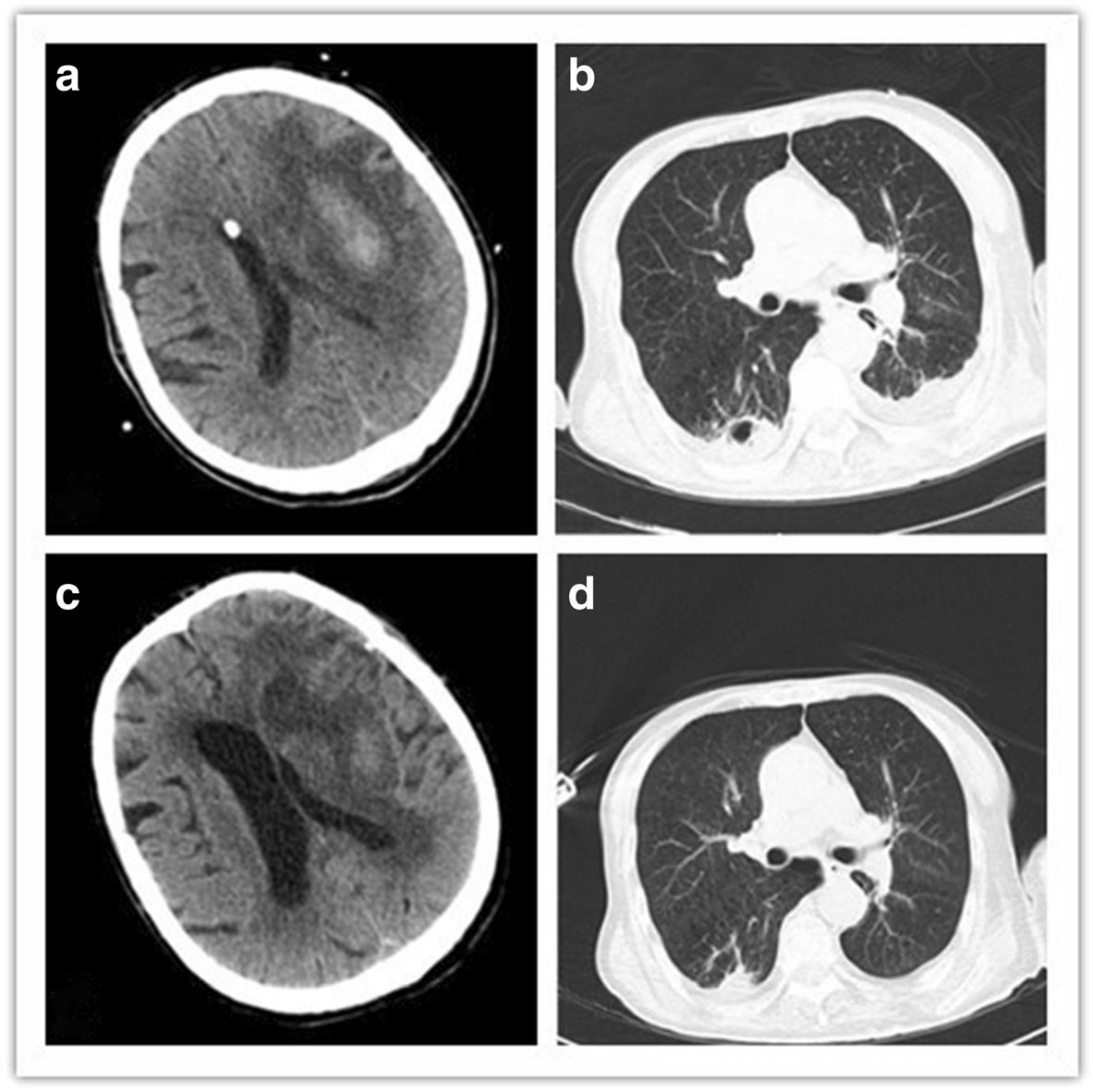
Computed tomography (CT) scans of the patient’s brain and lung during hospitalization. a. Cerebral CT scan revealed an extensive left hematoma in the temporal region of the hemispheres and moderate lateral ventricle enlargement with a drainage tube in it. The hematoma was surrounded by brain edema, narrowed gyri and the right–shifted cranial midline. b. Thoracic CT scan (lung windows) revealed a cavity with a wall of 7-mm thick surrounded by patchy shadowing in the right lower lobe. c. After 30 days of therapy, resolving brain swelling was seen in the CT scan with decreased edema. d. After 30 days of therapy, thoracic CT scan (lung window) revealed that the area of cavitation had decreased substantially
